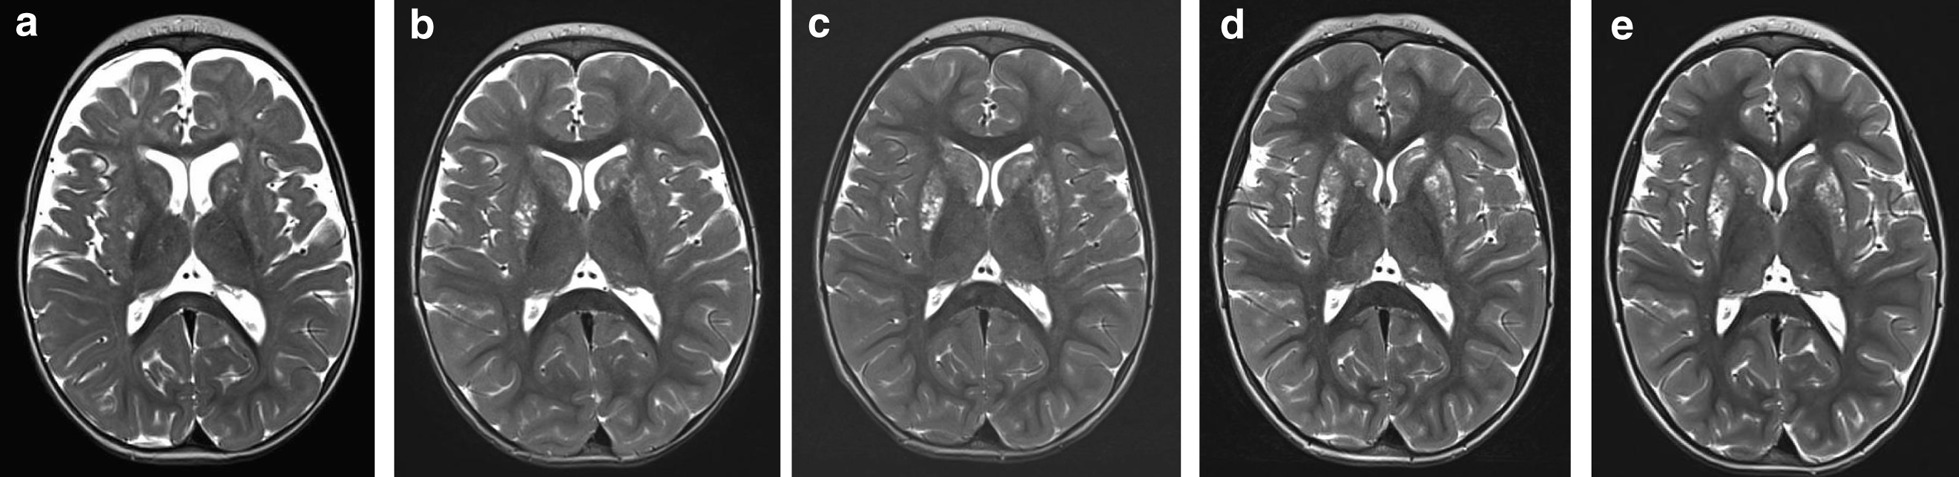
Longitudinal brain MRI findings. Brain MRI T2-weighted sections before living transplantation (a), and at one (b), two (c), three (d) and five (e) years of follow-up. Before liver transplantation, fronto-temporal atrophy and symmetric hyperintense lesions involving the putamina and the caudate nuclei were detectable (a). After liver transplantation, improvement of cerebral atrophy with no others new basal ganglia lesions occurred (b–e). A slight progression of basal ganglia lesions, especially at the left putaminal level, was observed between the 1st and the 3rd year (b–d), and then stabilized (d–e)
